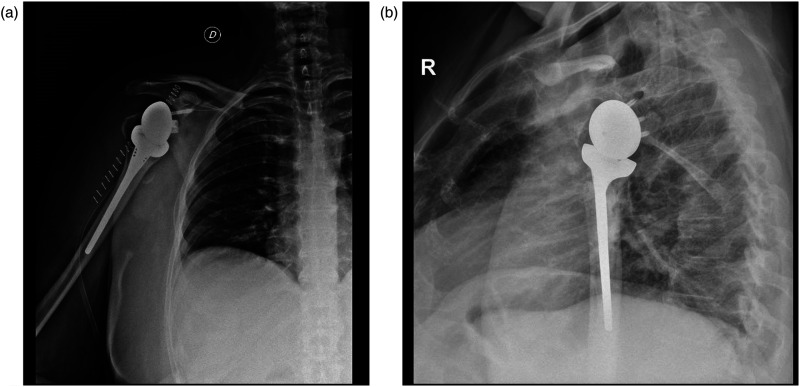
75-year-old male who underwent reverse shoulder arthroplasty after a complex proximal humerus fracture: anteroposterior (A) and transthoracic (B) radiographs immediately after surgery.
